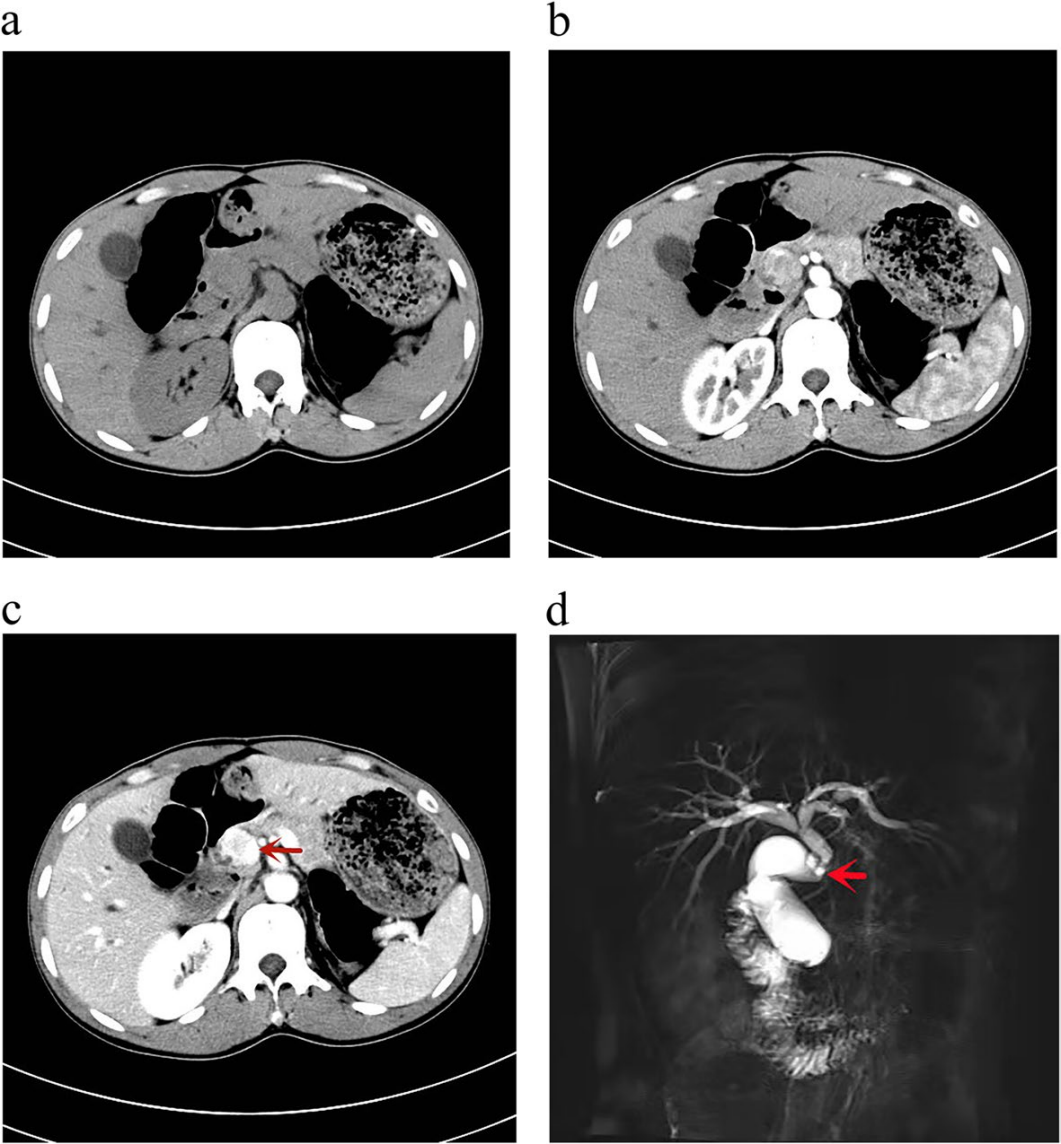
a Axial pre-contrast CT image. b axial post-contrast CT image in arterial phase. c Axial post-contrast CT image in portal venous image in delayed phase shows a heterogeneously enhancing mass at common bile duct (CBD) [red arrow in (c)]. d MRI image shows diffuse dilatation of the intrahepatic biliary radicles and proximal common bile duct (CBD) with narrowing noted at the middle of the CBD
